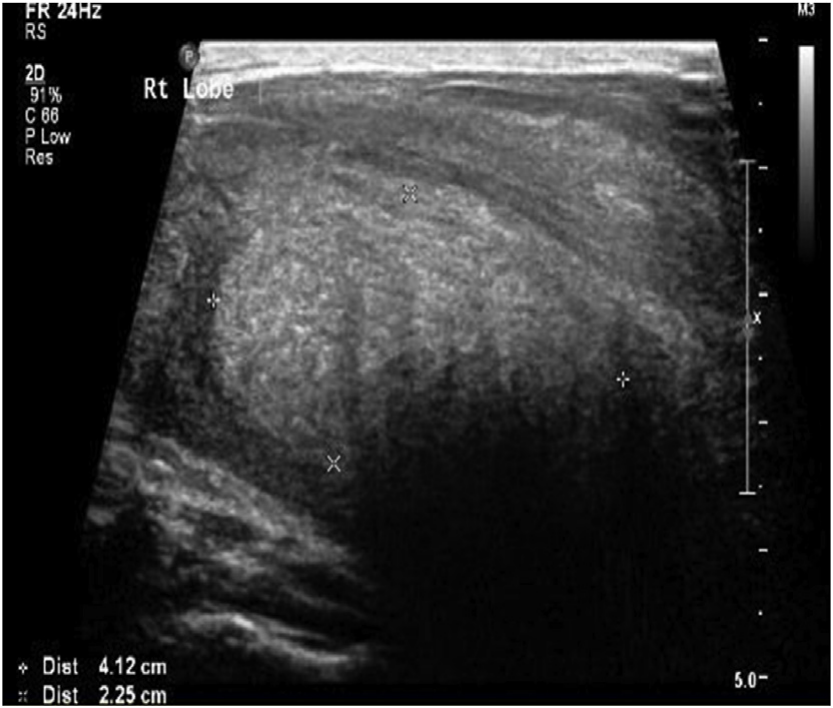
Ultrasound scan of the neck revealed Heterogeneous parenchymal echotexture is seen of both the thyroid lobes with multiple heterogeneous nodules seen. Couples of subcentimeter lymph nodes are seen in the bilateral cervical recesses.
